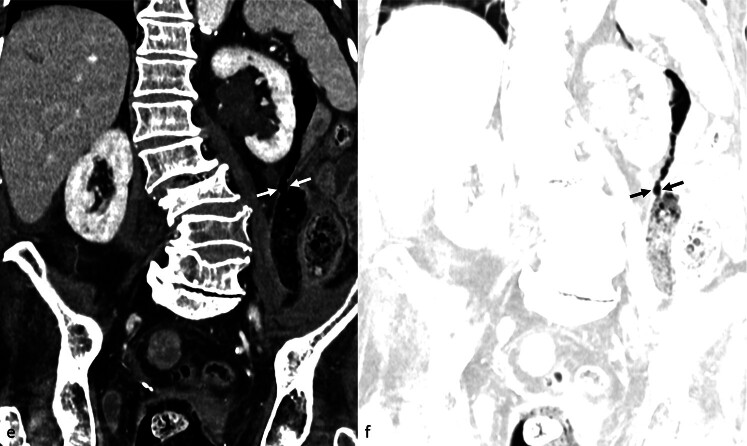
Coronal CT images of the abdomen. The site of perforation (arrows) in the descending colon is clearly visualized, with surrounding retroperitoneal gas extending along the left paracolic gutter.
